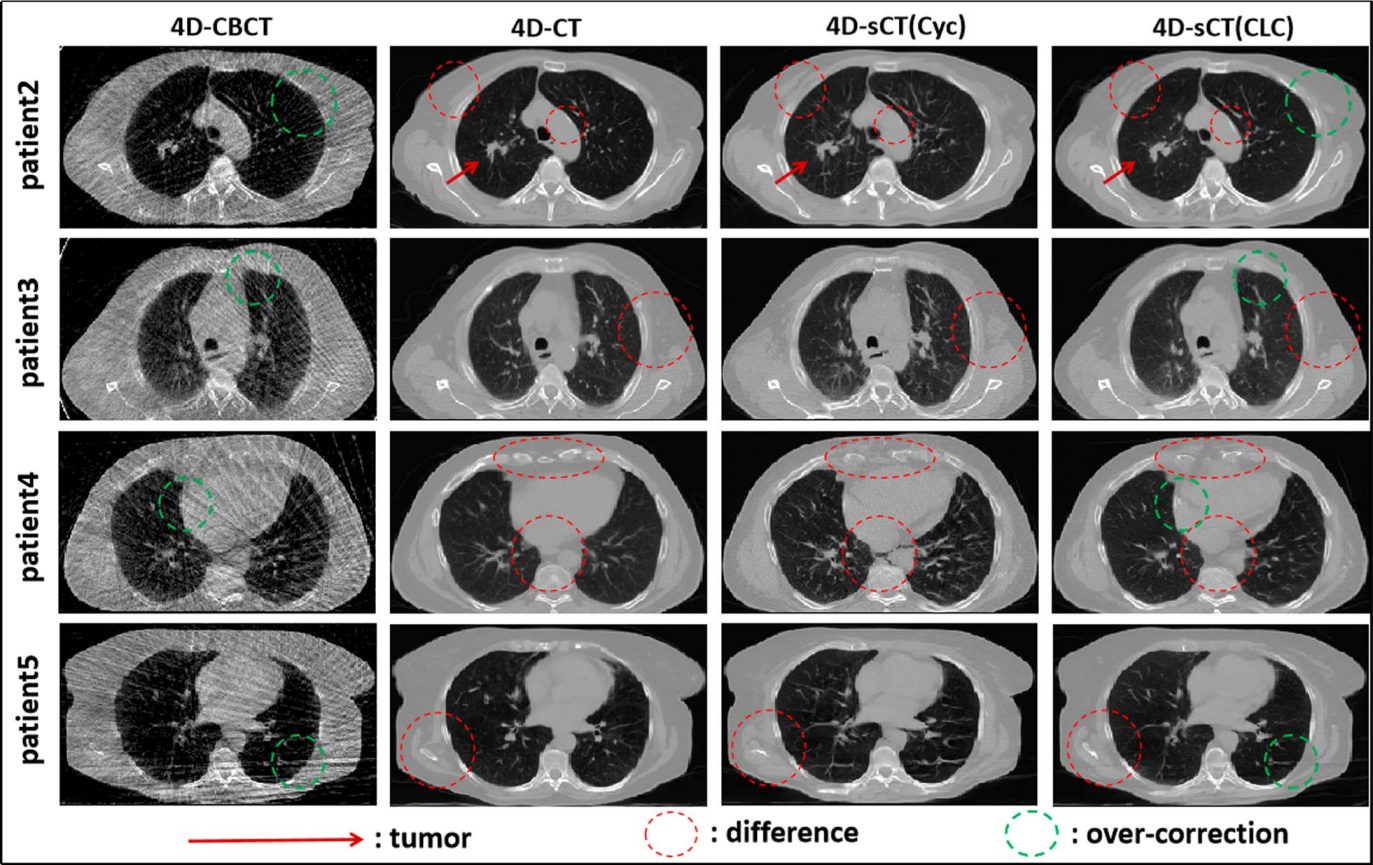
Structural images of four test patients. The four columns represent 4D-CBCT, 4D-CT,4D-sCT(Cyc), and 4D-sCT(CLC) images. All images are displayed at the same window width and window level
